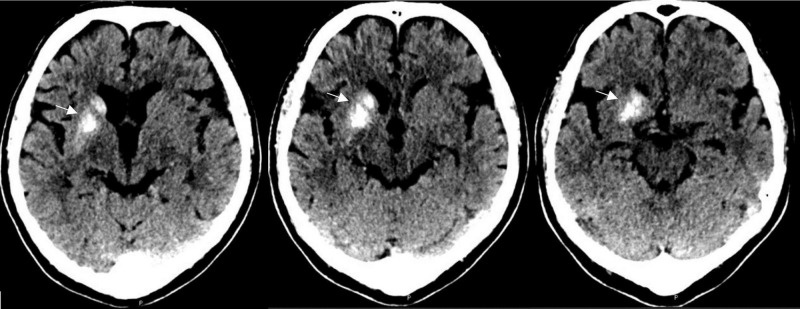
Computed tomography (CT) of the head without contrast showing unilateral putamen and caudate calcification sparing the anterior limb of the internal capsule
